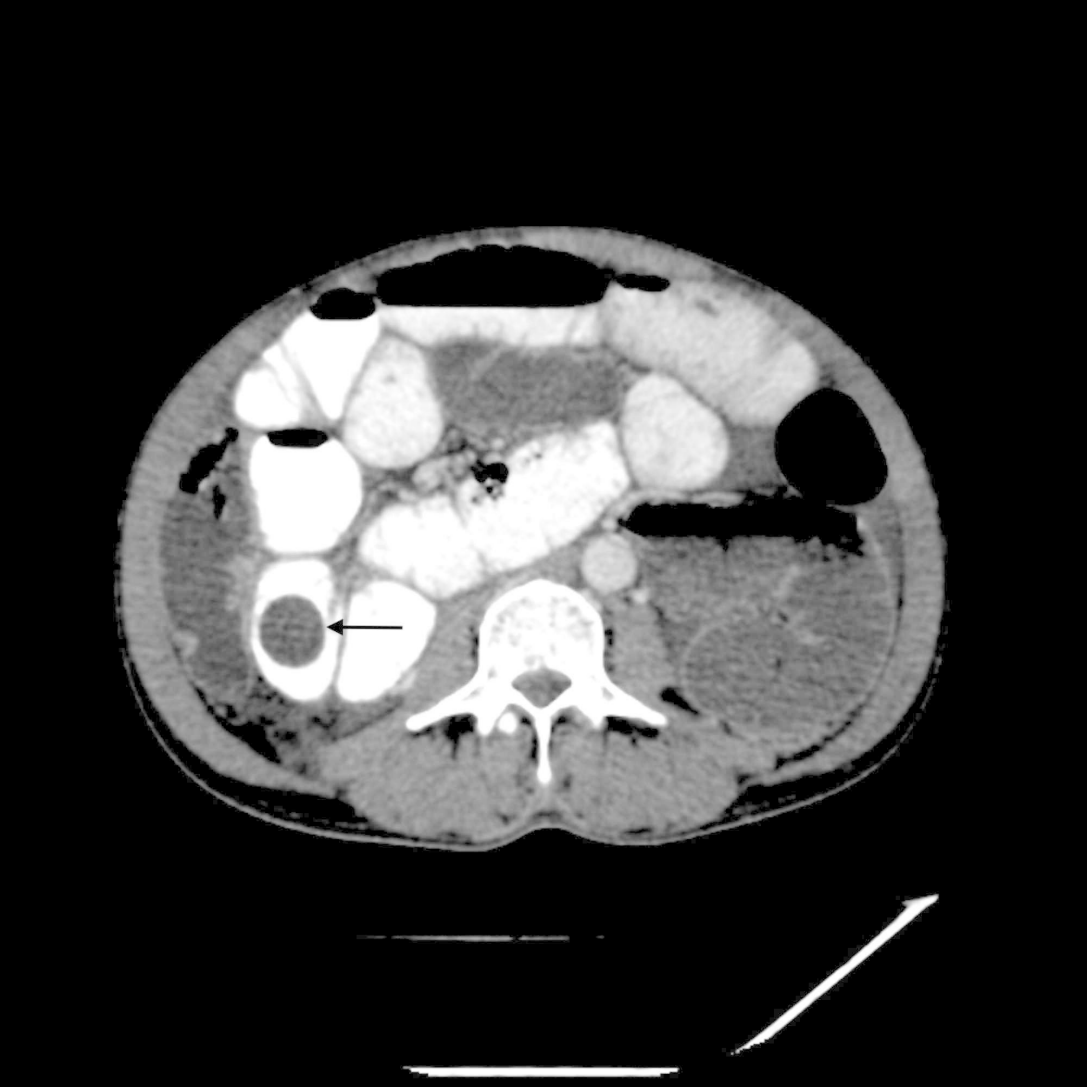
Axial CT image showing cyst within the jejunum. Black arrow depicts cyst within the jejunum.
